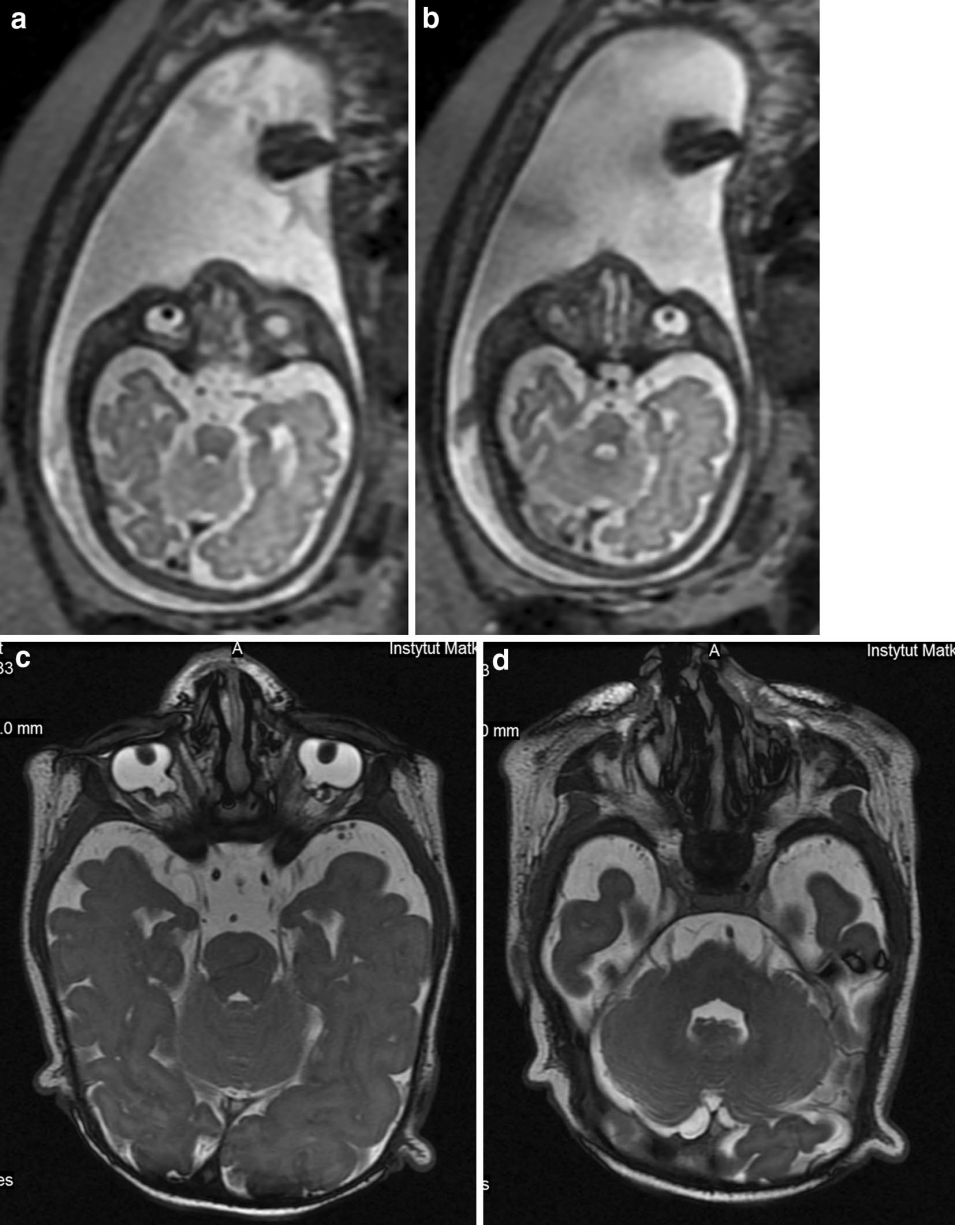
Case no. 14. a,b Fetal MRI. T2-weighted images, axial plane. Only deformation of the eye bulbs can be appreciated on these images. c Postnatal MRI. T2-weighted image, axial plane. Bilateral coloboma. d Postnatal MRI. T2-weighted image, axial plane. On the *left side* cranial nerves V, VII and VIII emerge together from the brain stem. On the *right* only cranial nerve V is visible in this section
